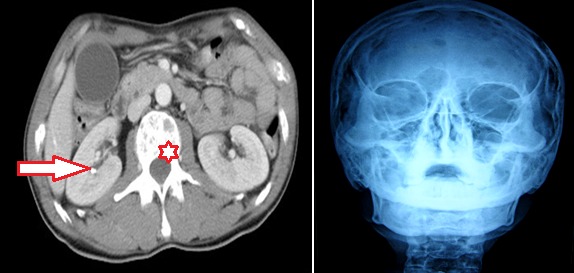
A) Coupe scanographique abdominale (L2): montrant une pancréatite stade C, des lésions ostéolytiques du corps vertébral (astérisque) et une lithiase calicielle (flèche); B) radiographie de crane de face montrant des lésions ostéolytiques à « l'emporte pièce »
